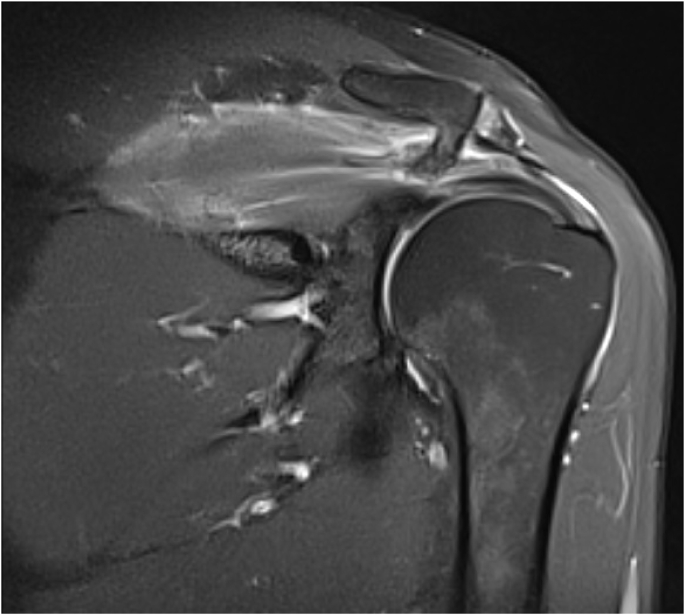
MRI revealed distal clavicle exostotic bone causing pressure effect on rotator cuff with cartilage cap.
